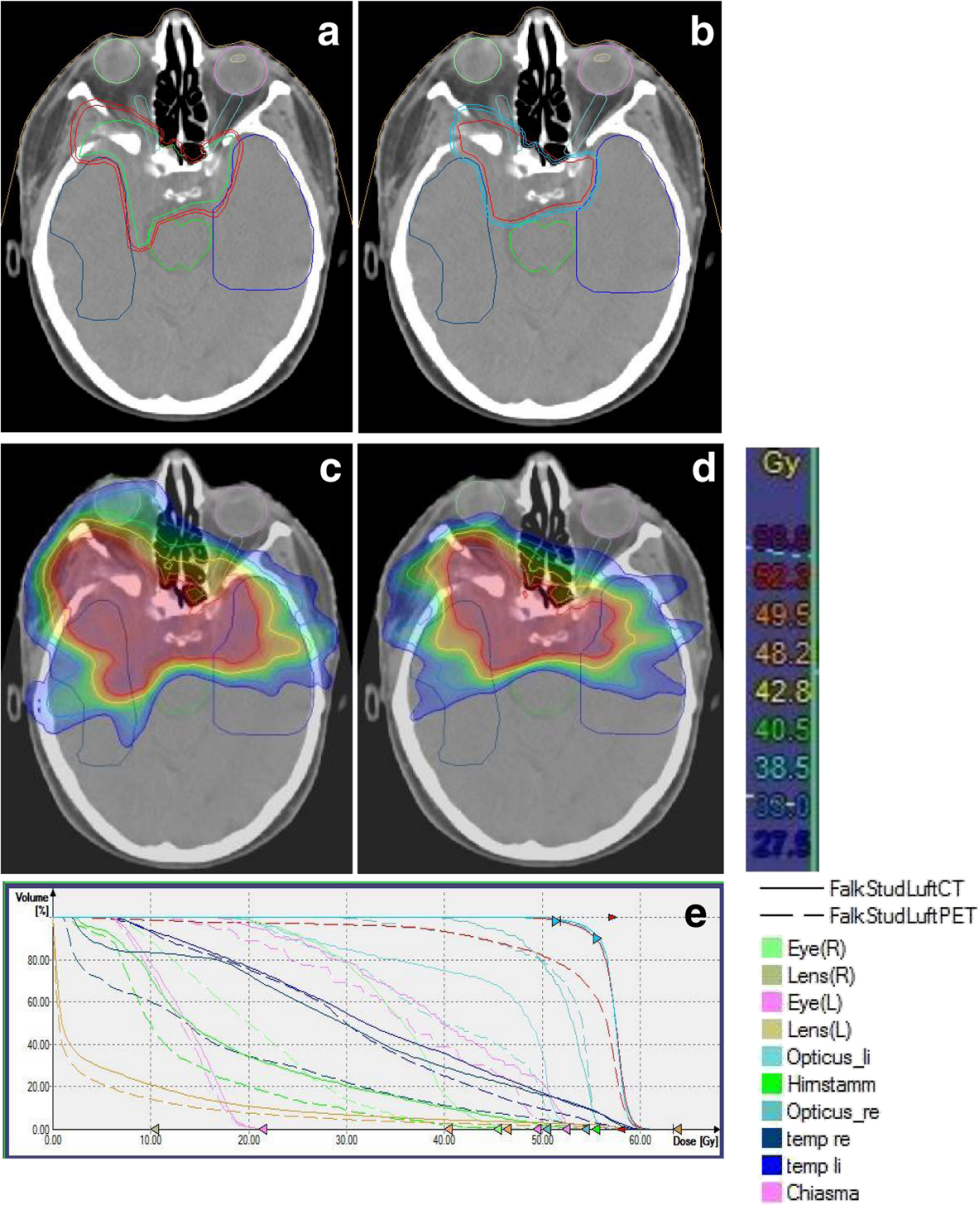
Target volume based on CT and MRI a and target volume based on CT, MRI as well as ^68^Ga-DOTATOC-PET b. Images c and d show the corresponding treatment plans with photon IMRT. Image e shows the Dose-Volume-Histogram comparing dose to the target and to OAR
